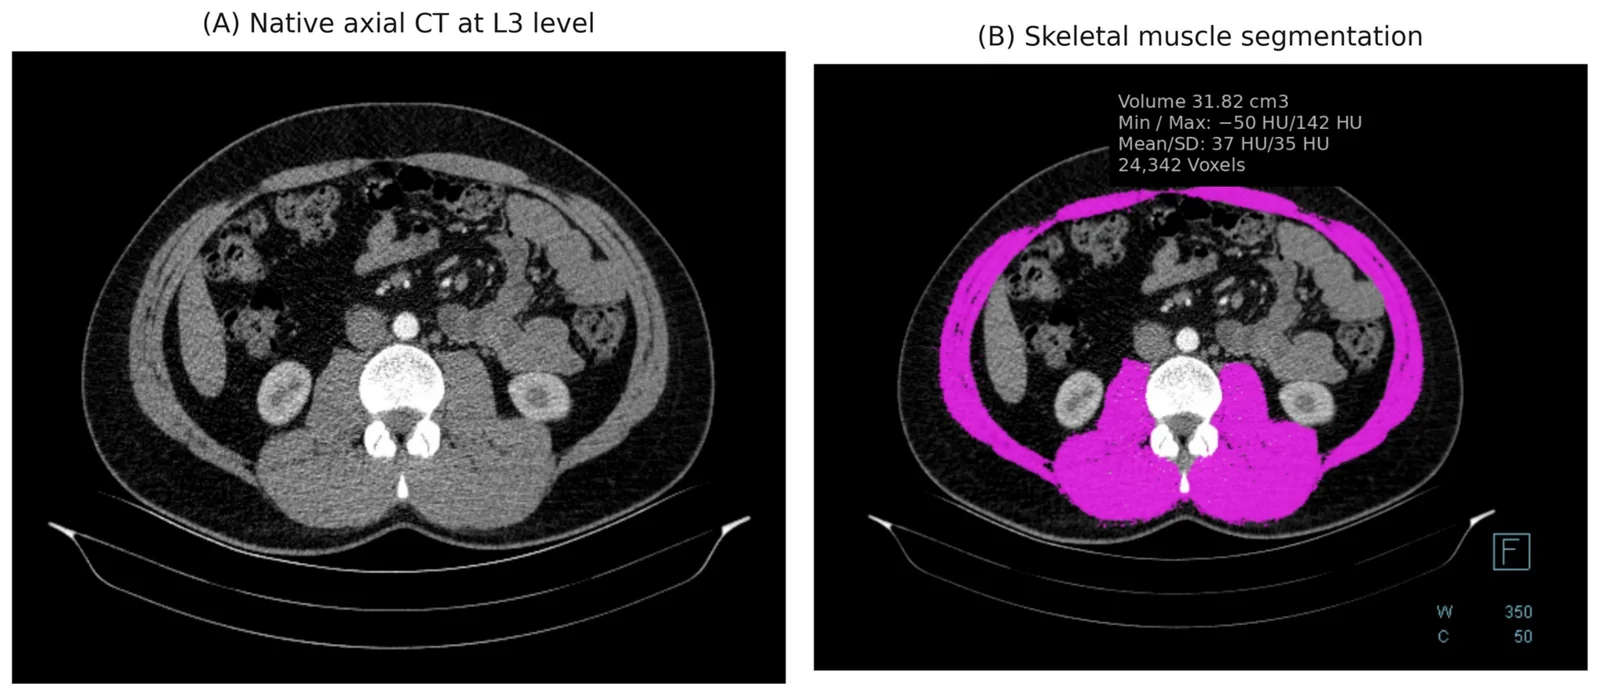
Representative skeletal muscle segmentation at the third lumbar vertebra. (A) Native axial computed tomography image at the level of the third lumbar vertebra. (B) Skeletal muscle compartments, including the psoas, paraspinal, and abdominal wall muscles, segmented with attenuation-based tissue identification within a Hounsfield Unit threshold range of −29 to +150 HU. The segmented muscle area was normalised to the height squared to obtain the skeletal muscle index.
